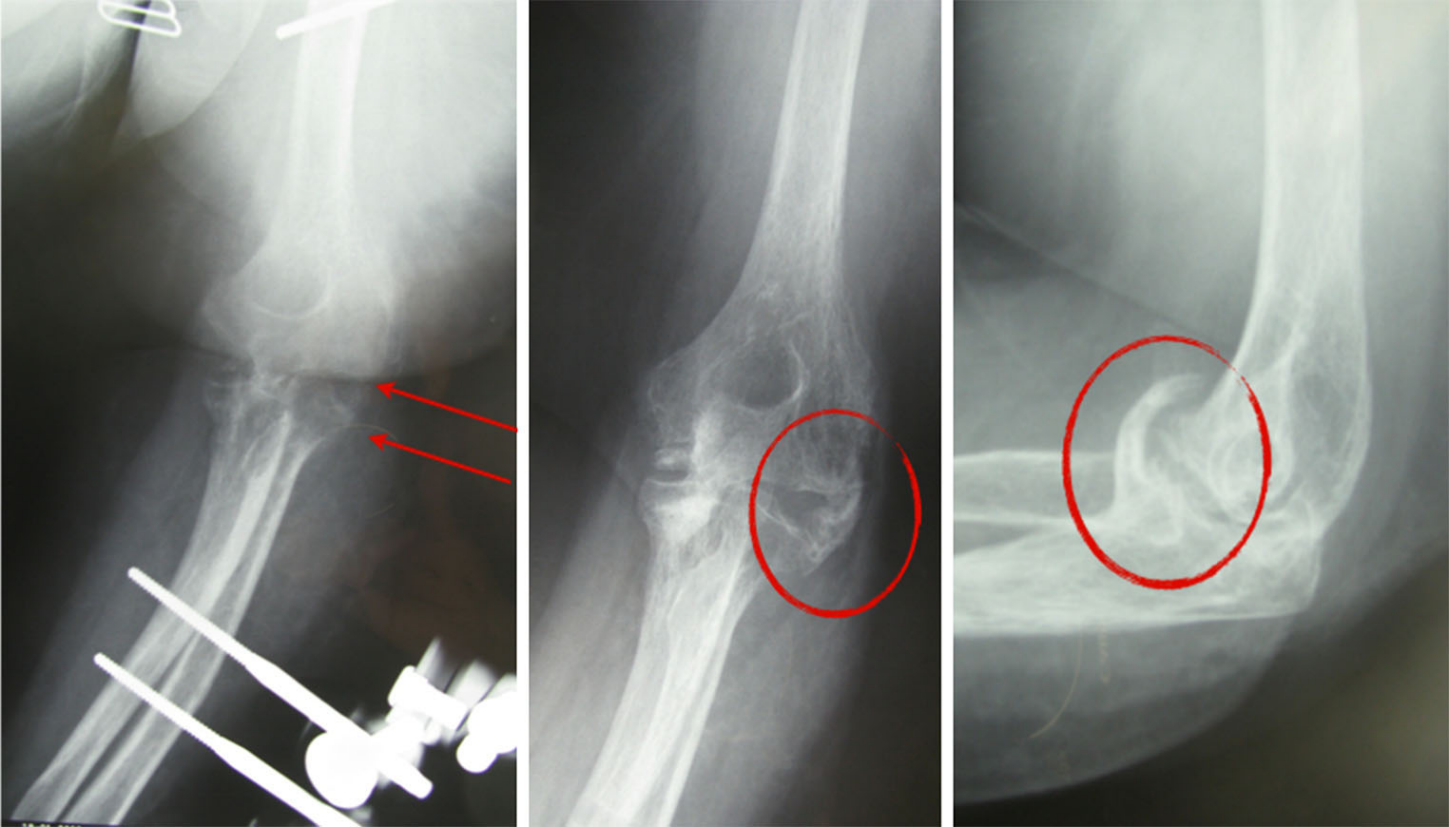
X-ray demonstrate bone metaplasia formation within the anterior capsule and collateral ligament complex
